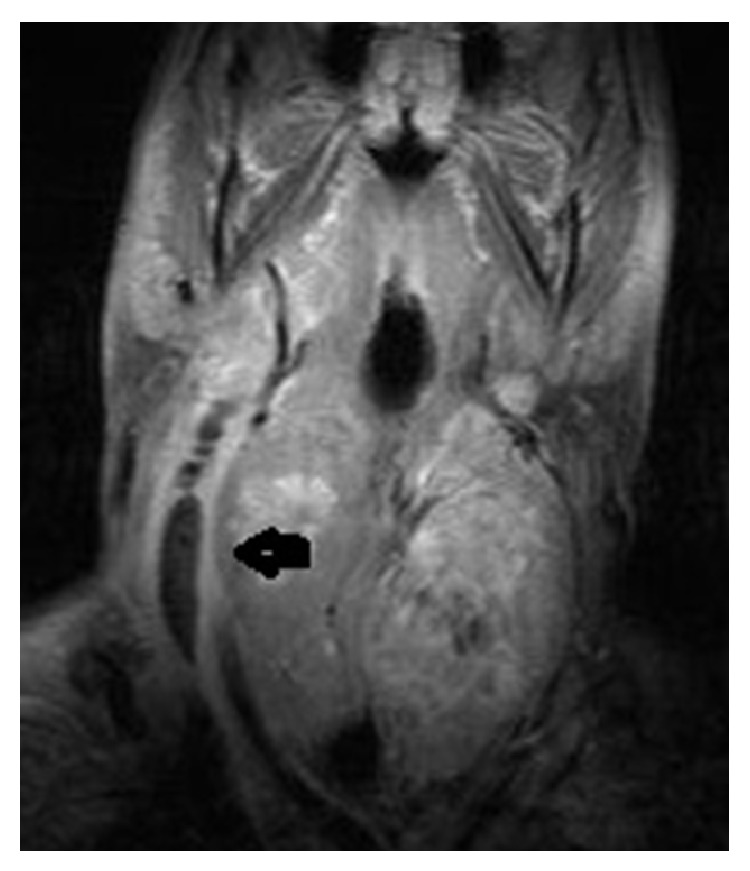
Neck MRI with contrast showed internal jugular venous distention with a thickened enhancing wall and filling defects.
